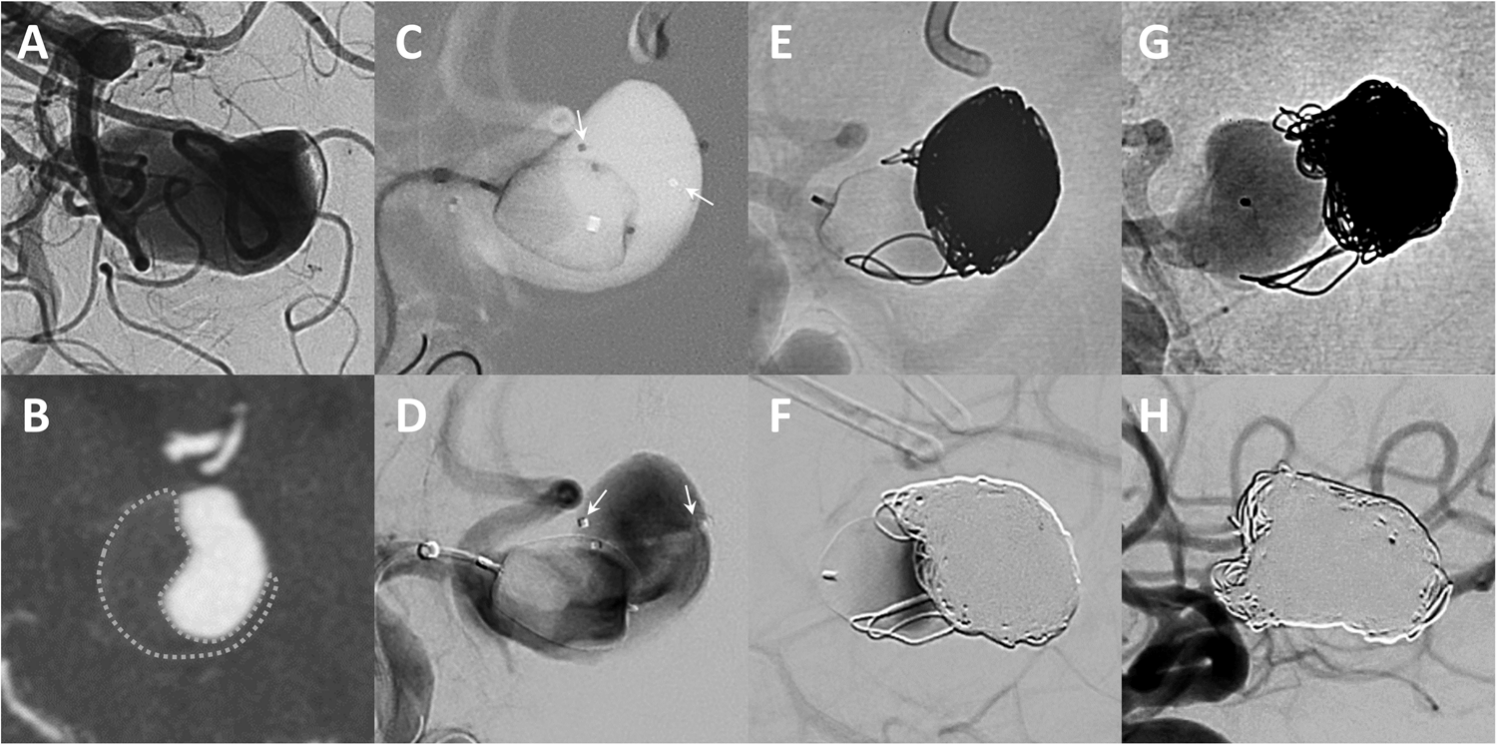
Digital subtraction angiography shows a giant ruptured aneurysm (dome width: 14 mm, height: 25 mm, neck width: 7.5 mm) of the anterior communicating artery (A). The aneurysm is partially thrombosed as shown on computed tomography angiography (B), the outline of the thrombus is highlighted with a dotted line in this image. As the aneurysm was ruptured, stent-assisted coiling was not chosen to avoid antiplatelet therapy. Instead, WEB-assisted coiling was chosen with the intention of placing the WEB at the base of the aneurysm to seal the aneurysm neck. With this intention, a WEB SLS 11 mm was deployed via a 0.033" microcatheter at the aneurysm base, and a second microcatheter (arrows) was introduced into the aneurysm sac using the Jailing technique for subsequent coil embolization (C: roadmap, D: subtracted images). The aneurysm dome was then embolized with 17 coils, resulting in an almost complete occlusion (E). Immediate control angiography shows incipient contrast retention within the WEB (F). However, 6-month follow-up angiography shows migration of the coils and WEB within the pre-existing aneurysm thrombus and both coil and WEB compaction resulting in extensive aneurysm recanalisation with a large aneurysm remnant (G). The aneurysm was then retreated with stent-assisted coiling to achieve complete occlusion (H).
